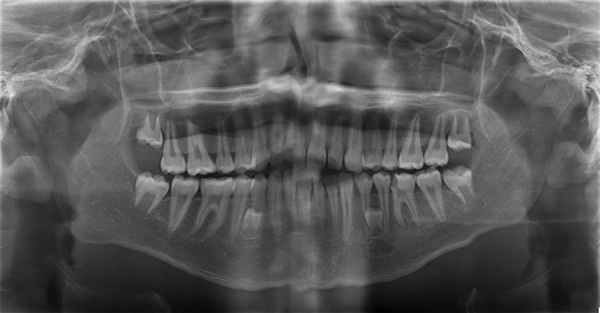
Orthopantomogram showing bilateral unerupted supplemental premolars in mandibular arch
